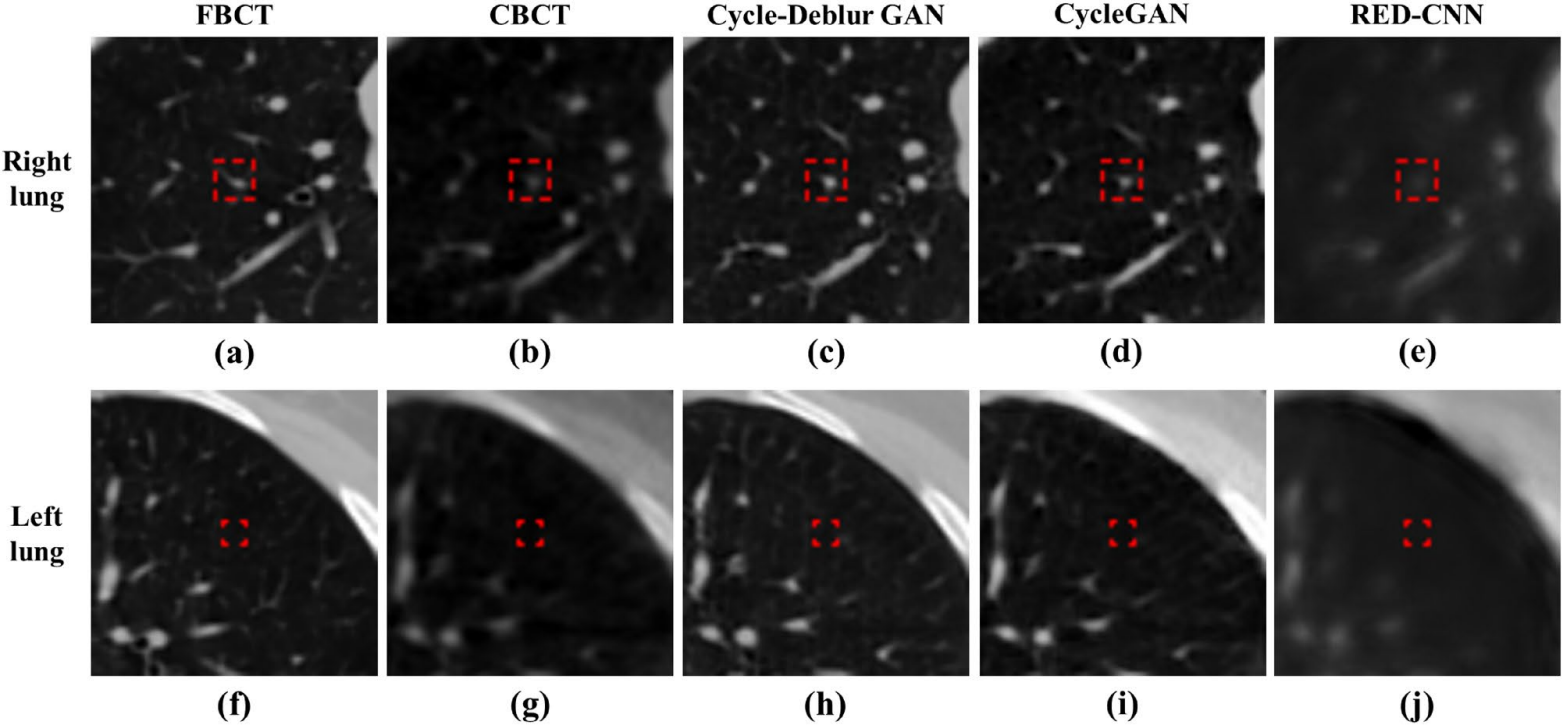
The right and left lung ROIs for quantitative evaluation are shown in (a,f): FBCT, (b,g): CBCT, (c,h): Cycle-Deblur GAN, (d,i): CycleGAN and (e,j): RED-CNN were performed by XVI software and PMOD software. (W = 1450, L = − 225 for all CT images).
